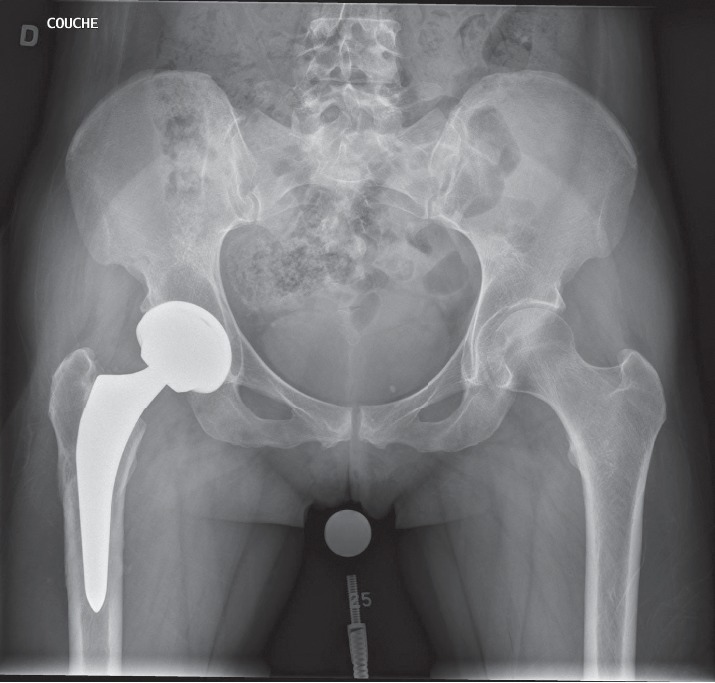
AP pelvic X-ray 6 weeks after right noncemented THA.
